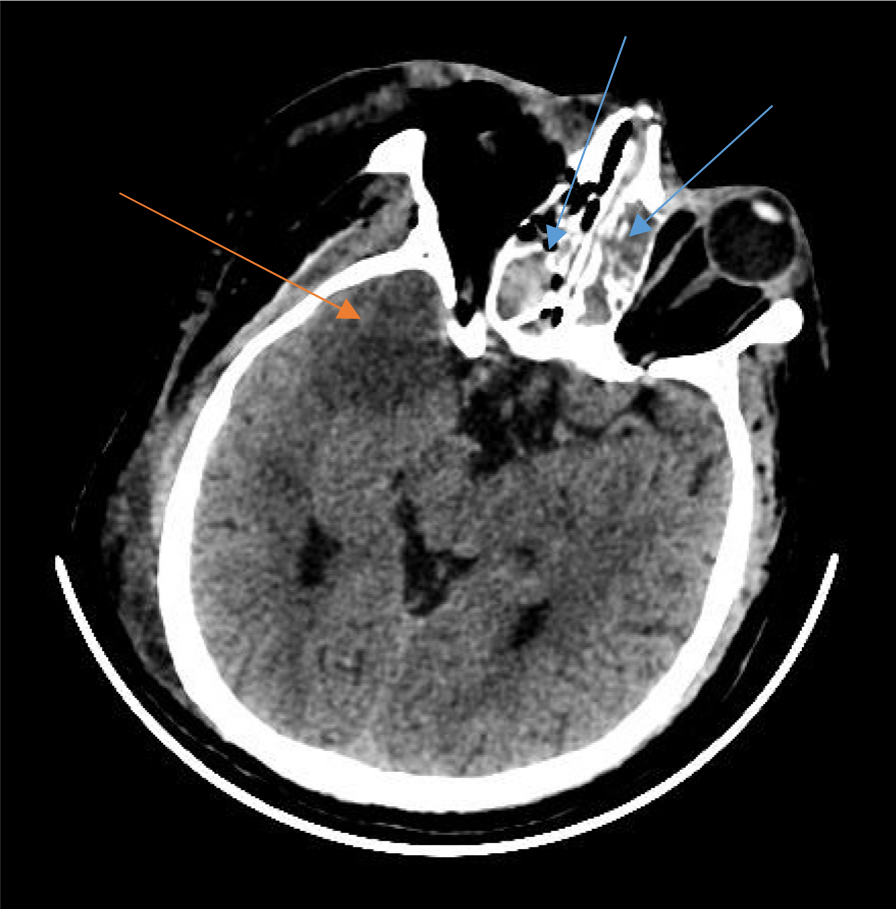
Axial section of plain CT of the brain in a patient with rhino-cerebral mucormycosis showing hyperdense mucosal thickening of bilateral ethmoid sinuses (blue arrows) and a wedge-shaped hypodense area in the right temporal lobe suggestive of acute infarct of the right middle cerebral artery territory (red arrow)
